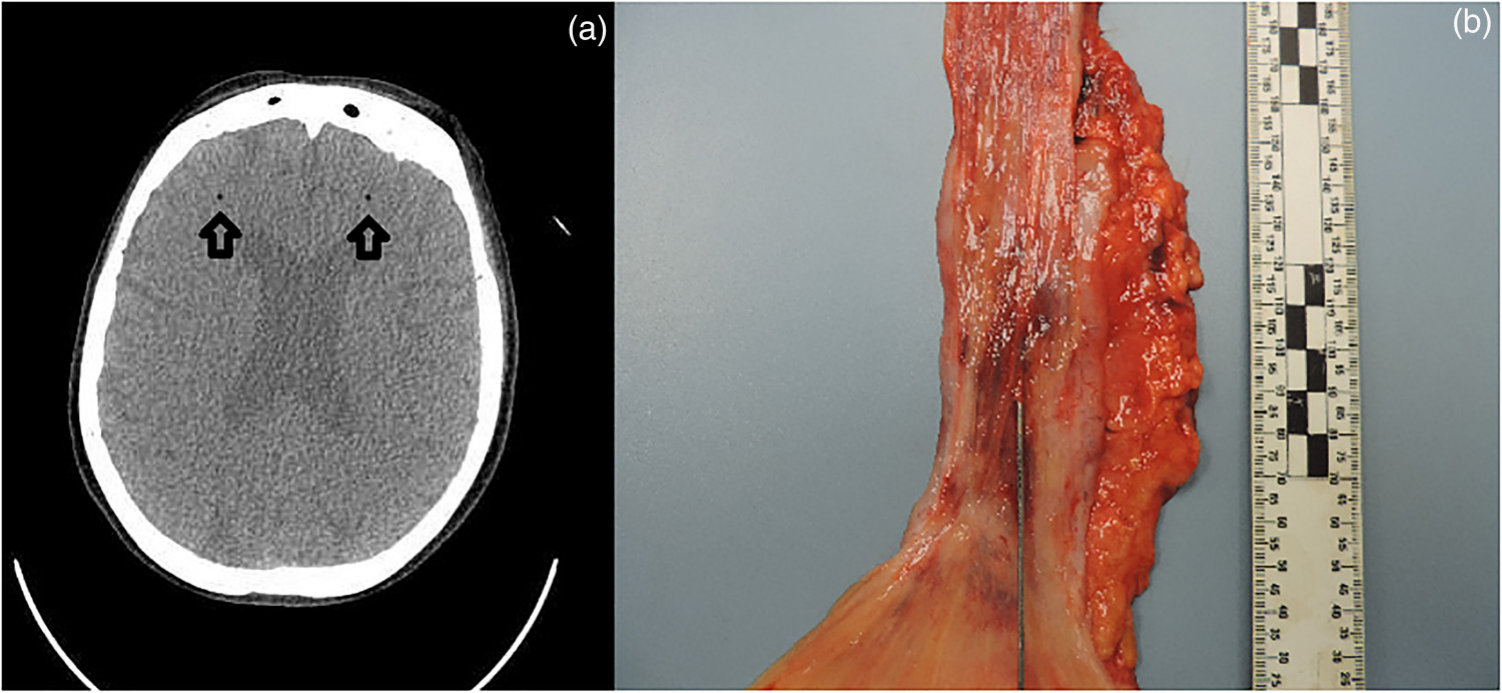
(a) Antemortem CT imaging of central nervous system air emboli (arrows), (b) Gross image of esophageal mucosal defect.
